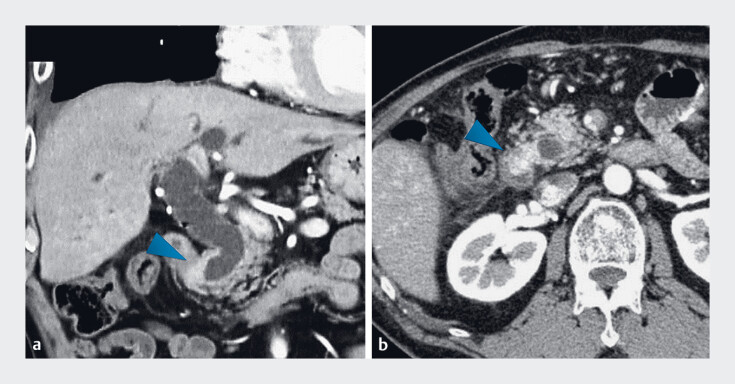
Computed tomography depicting a tumor in the distal bile duct (arrowheads). The images revealed stricture at the tumor site accompanied by proximal bile duct dilation.
